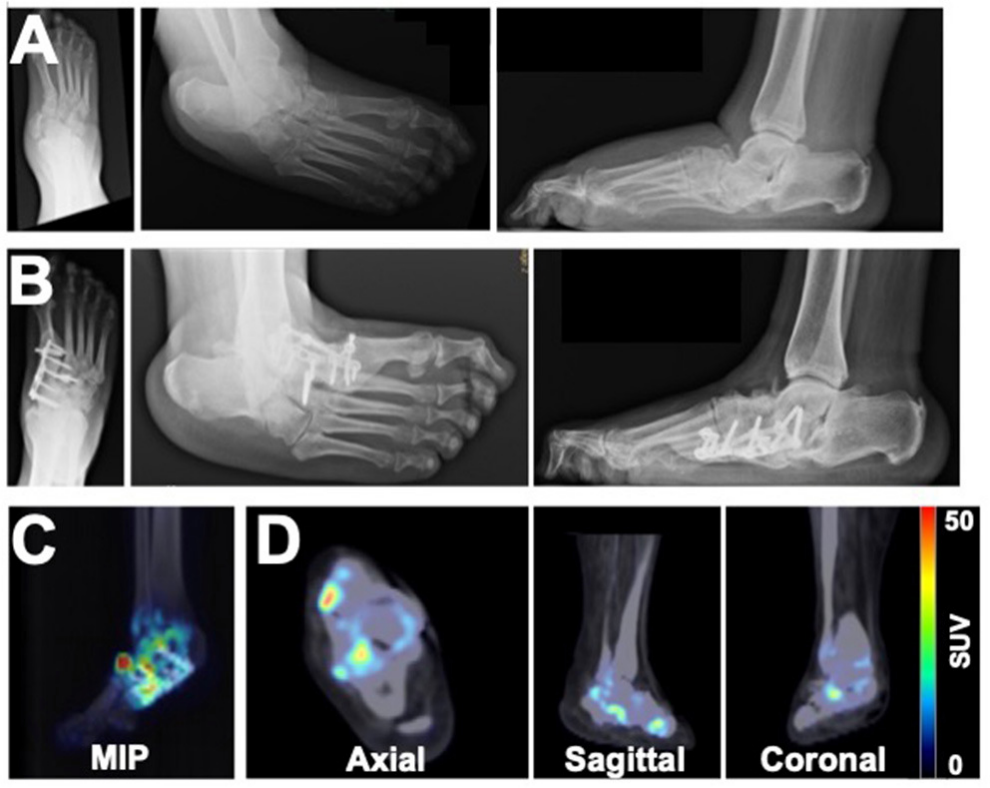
Imaging assessment of a 49-year old female patient with a history of right foot Charcot deformity. X-rays were acquired at (A) the time of navicular bone removal and (B) 5 years after surgical reconstruction of the foot. (C) MIP and (D) PET/CT images acquired 5 years after surgery revealed heterogeneous boney uptake of ^18^F-NaF and suggested potential for ongoing remodeling of the foot.
